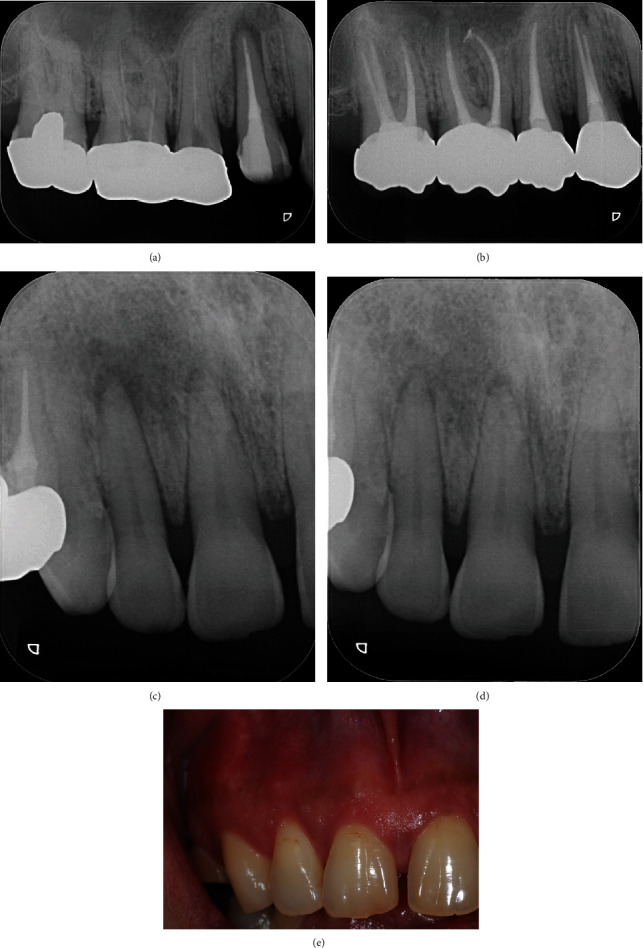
Postoperative intraoral radiographs and photograph. Intraoral radiograph of the maxillary first premolar just after root canal filling (a). Intraoral periapical radiograph of the maxillary right central and lateral incisors after 6-month period from the retreatment of only the first premolar; the radiolucent regions tended to heal (c). Intraoral periapical radiograph of the maxillary first premolar and incisors after a three-year period: the radiolucent region of the premolar was healing. The retreatment of the root canal of the second premolar and first and second molars were realized (b), and the radiolucent regions of incisors had almost disappeared (d). Intraoral photograph of right central and lateral incisor after three years (e).
